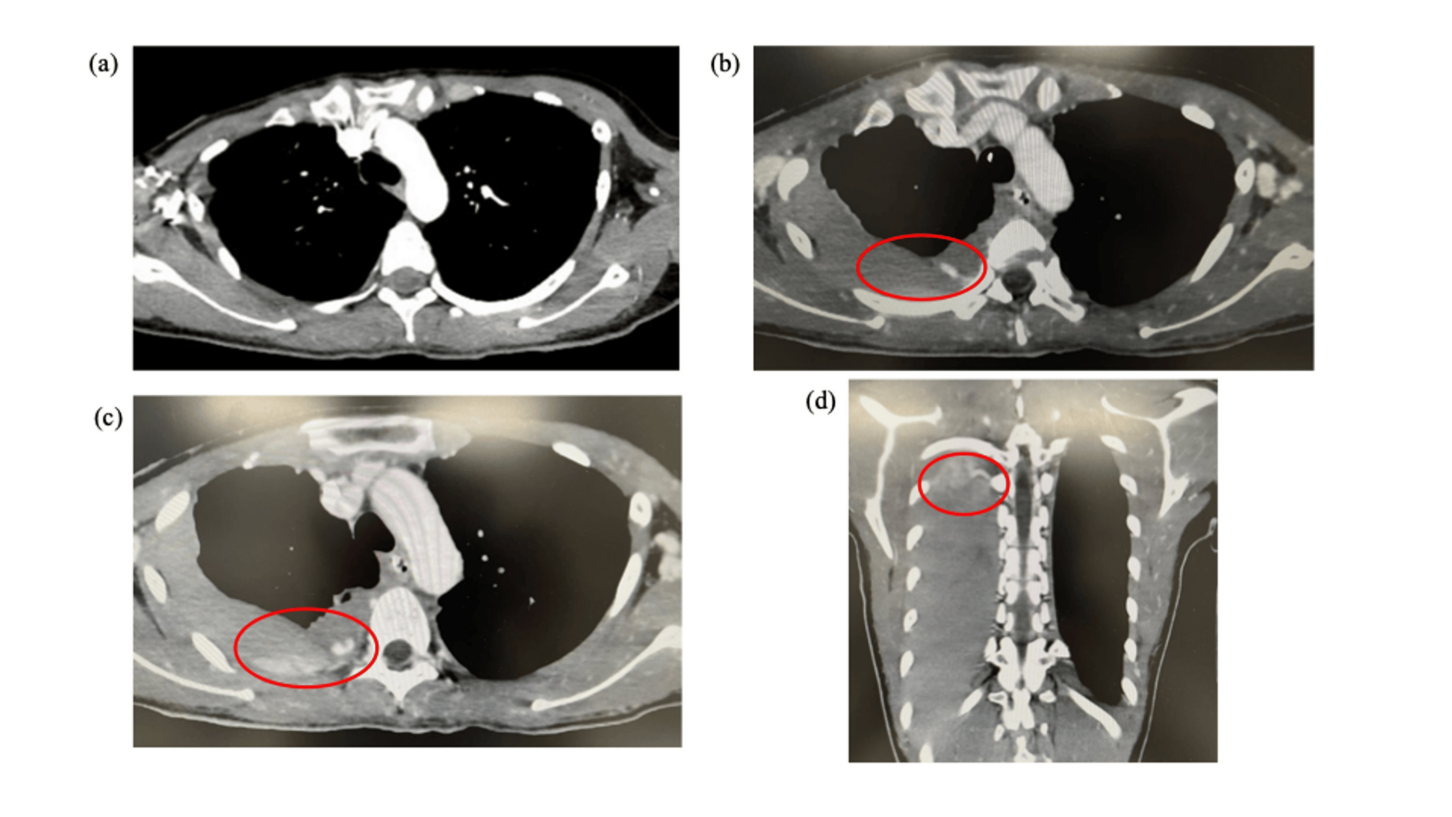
Comparison between the initial and post-treatment computed tomography performed 3 h apart (a) The initial computed tomography revealed no obvious pathological findings (b), (c), (d) Post-treatment computed tomography revealed a massive right-sided hemothorax with active contrast extravasation (red circle) that had not been evident on the initial scan, prompting emergency tube thoracostomy followed by repeat embolization.
